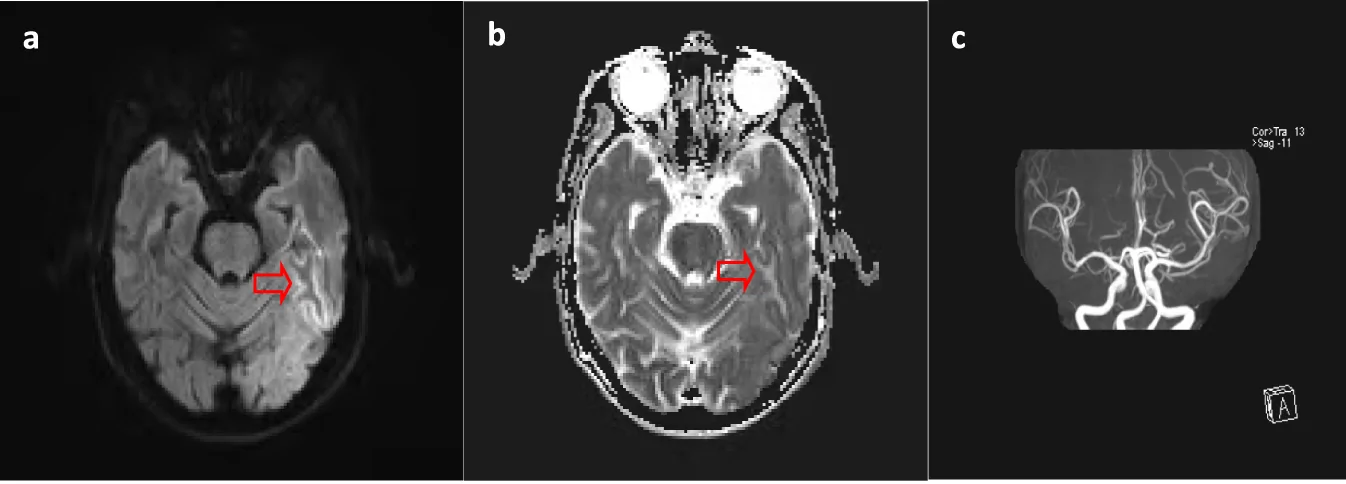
21 November 2022 MRI: (a,b) Lacey like DWI high signal and ADC low signal are observed in the left temporal occipital parietal lobe; (c) MRA did not show cerebral artery stenosis in the lesion area, which does not conform to vascular distribution.
